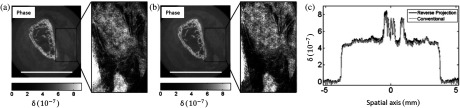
Phase tomograms of a chicken bone sample imaged with a conventional x-ray source and retrieved with the (a) reverse projection and (b) conventional EI retrieval algorithms. Scale bars indicate 5 mm. Quantitative values are compared in panel (c), where profiles extracted along the central horizontal lines in the respective tomograms are plotted together. Adapted with permission from IOP Publishing: Journal of Physics D: Applied Physics, Ref. 46, Copyright 2016.
